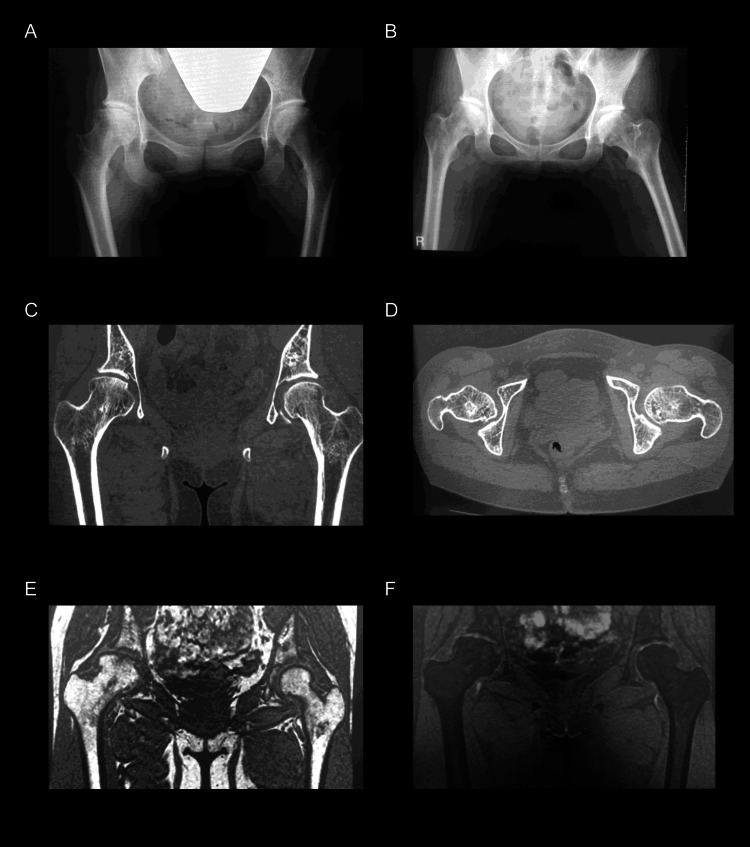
Imaging evaluation findings A) Frontal radiography of the hip joint; B) Frontal radiography of the hip joint during abduction. No impingement of the acetabulum is observed during abduction of the right femoral head; C) Coronal computed tomography of the hip joint; D) Transverse computed tomography of the hip joint is shown. Bone sclerosis is observed in the right femoral head, and the necrotic bone is remodeled; E) Coronal T1-weighted magnetic resonance imaging; F) Coronal fat-suppressed magnetic resonance imaging reveals no signs of inflammation.
